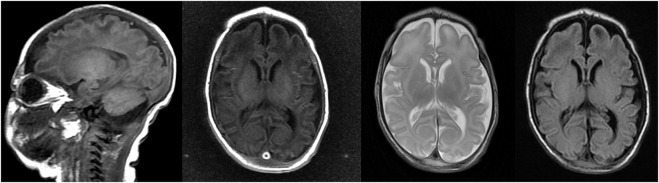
Cranial MRI. From left to right: T1 FLAIR, T2 plain scan, T2 FLAIR. Bilateral frontoparietal white matter shows patchy slightly long T1 and long T2 signals on T1WI and T2WI sequences, and hypointense signals on FLAIR sequence. The left lateral ventricle is slightly enlarged, with widened bilateral sylvian fissures, anterior longitudinal fissure, and frontal extracerebral spaces. The corpus callosum is relatively thin.
